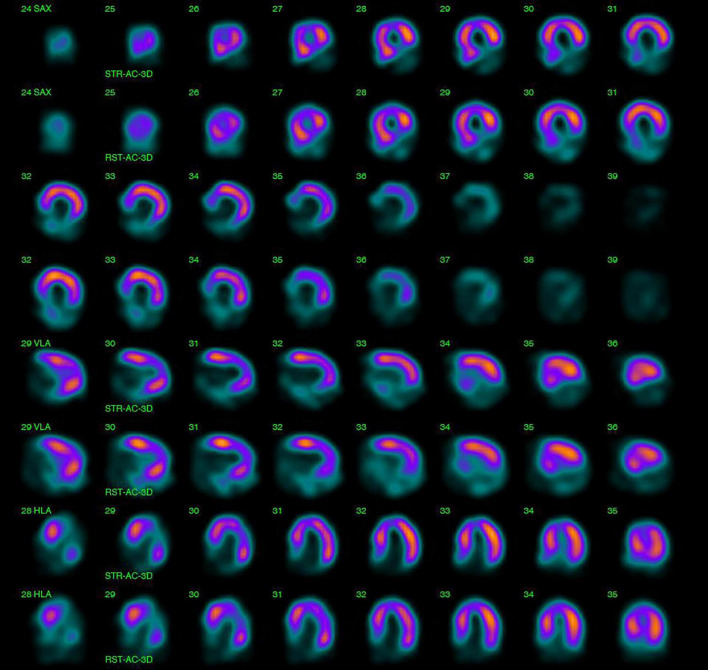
Single-photon emission computed tomography (SPECT) for the assessment of myocardial ischemia and viability. 47-year-old male with a history of Hodgkin lymphoma, treated with radiotherapy and chemotherapy, was diagnosed with regional wall motion abnormalities in the inferior left ventricular wall during regular echocardiographic surveillance and was referred for SPECT. A fixed perfusion defect in the basal and mid inferoseptal, inferior and inferolateral left ventricular segments was found on SPECT with a summed stress and rest score equal to 16 (summed difference score 0). Findings were consisted with a silent myocardial infarction, characterized by non-viable myocardium in the right coronary artery perfusion territory.
